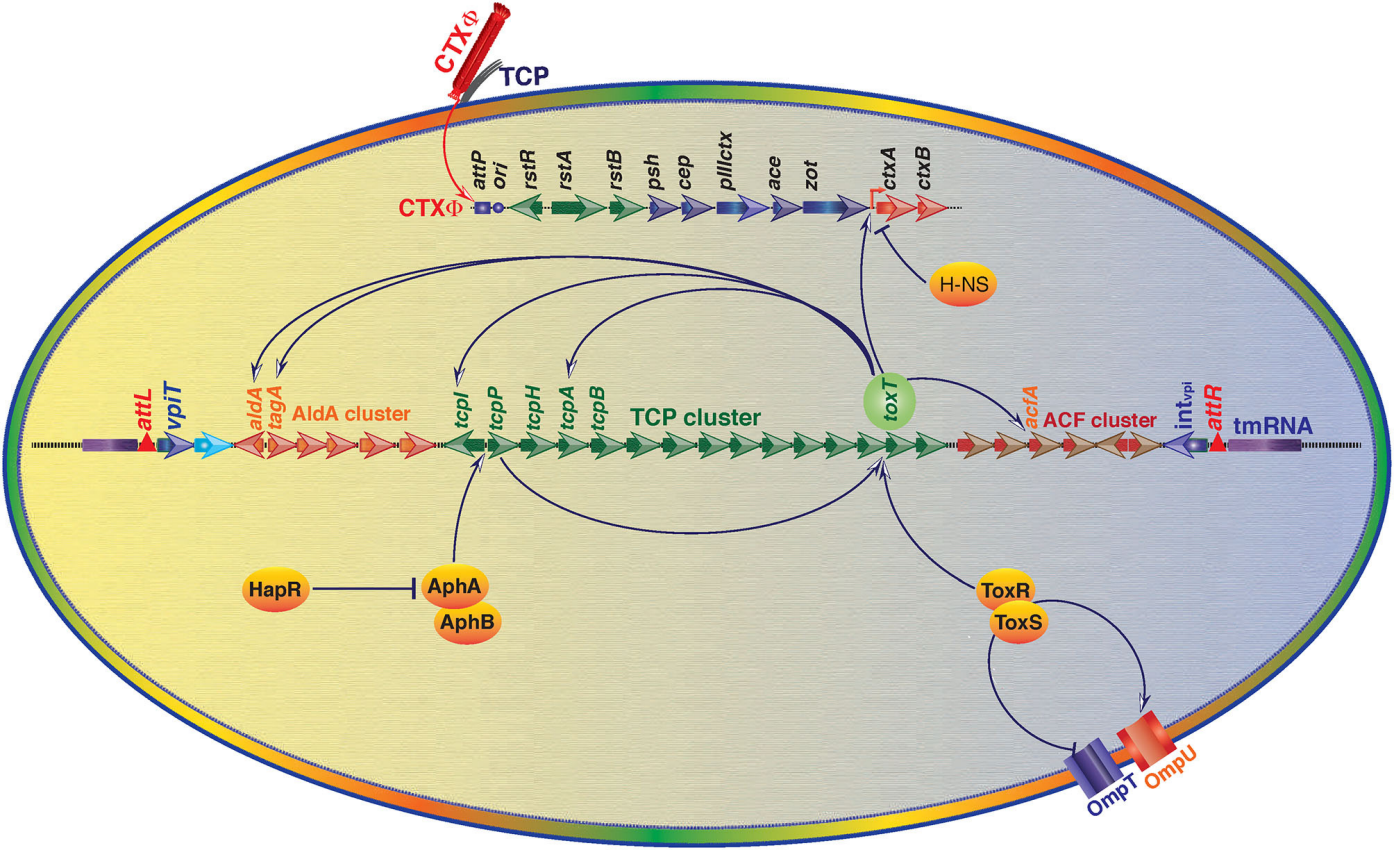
An overview of regulation of *V. cholerae* virulence gene expression through Vibrio Pathogenicity Island 1 (VPI-1) and core genome encoded functions. Transcriptional factor ToxT is the key positive regulator of cholera toxin (CT) encoding genes *ctxA* and *ctxB*. ToxT also positively modulates expression of toxin co-regulated pilus (TCP) genes and several other genes located in the VPI-1. ToxR-ToxS and AphA-AphB proteins positively regulate expression of *toxT*. ToxR-ToxS proteins also modulate expression of outer membrane proteins OmpU and OmpT. Histone-like nucleoid-structuring (H-NS) protein negatively regulates expression of CT. Transcriptional regulator HapR negatively regulates expression of AphA and AphB encoding gene expression. Positive regulation is depicted by arrowhead and T head depicts negative regulation.
